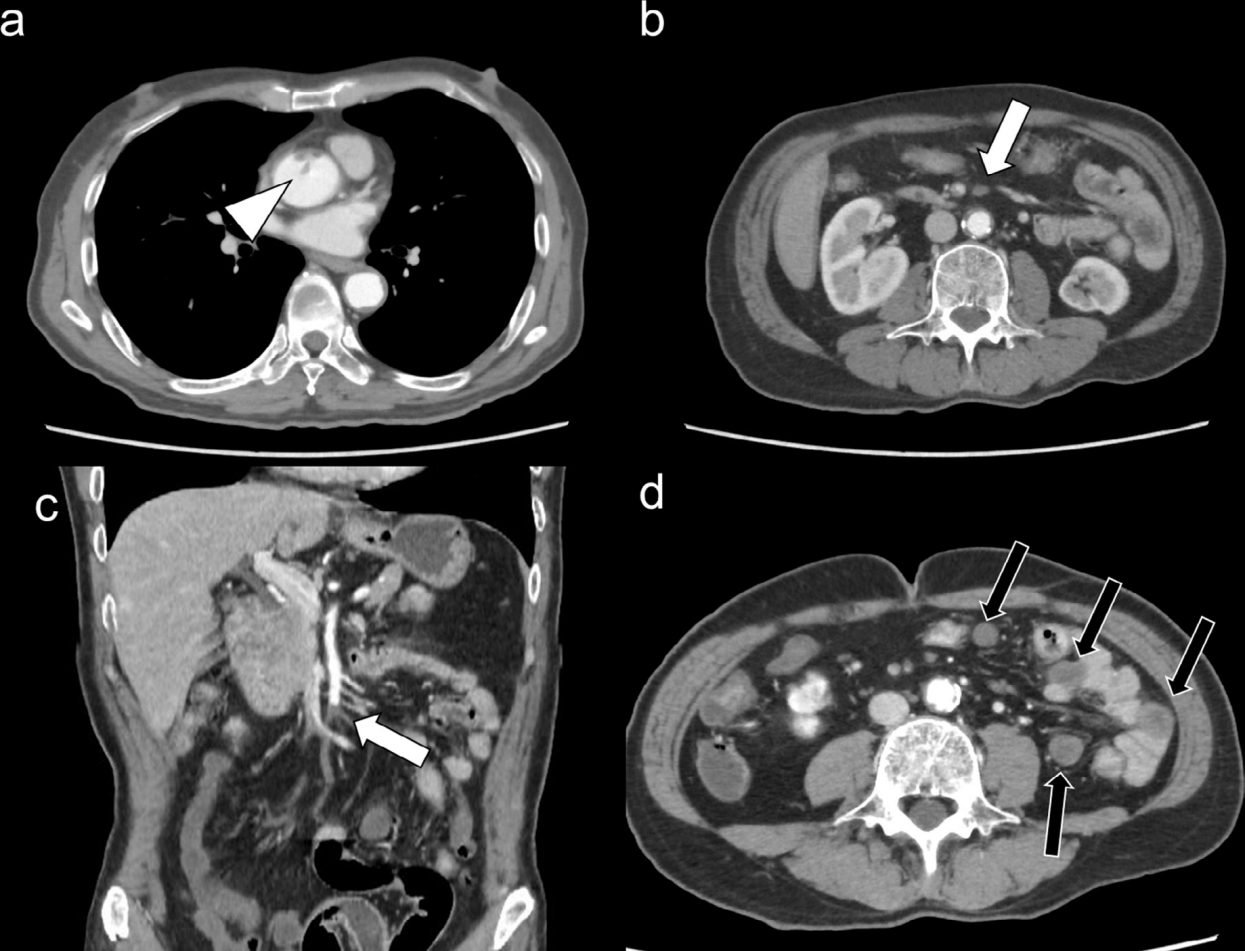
Contrast-enhanced computed tomography shows an aortic thrombus in the ascending aorta (a) and SMA embolism (b: axial view, c: coronal view). The arrowhead indicates a floating thrombus and white arrows indicate SMA embolism. Contrast-enhanced computed tomography also showed decreased contrast enhancement in the small intestine (d: black arrows).
